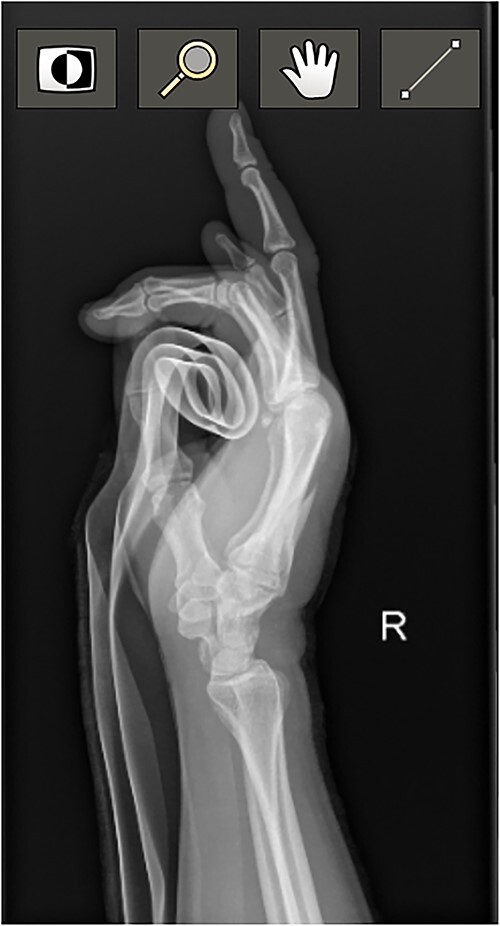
Diagnostic Radiograph 1.
